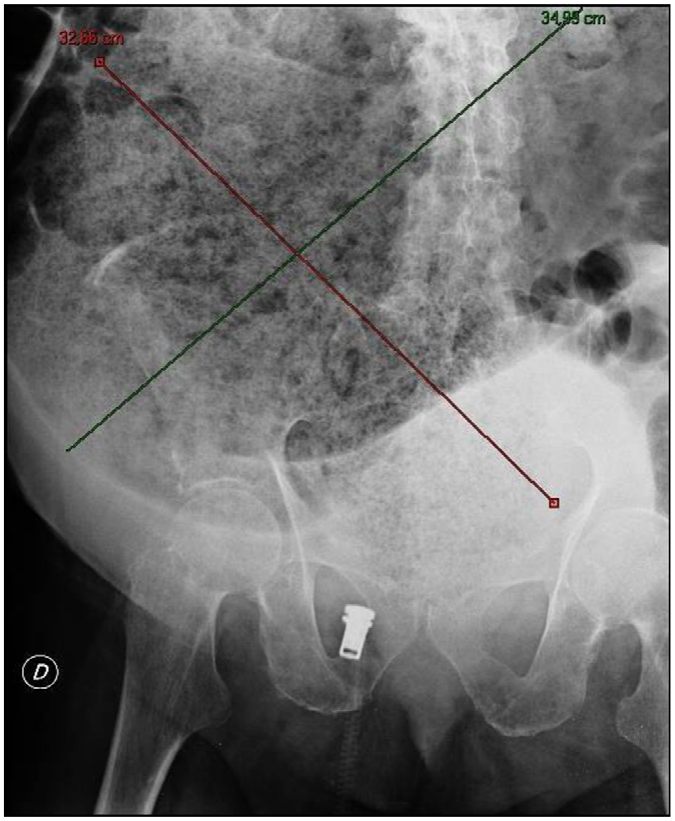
Initial plain abdominal X-ray done in emergency room showing a huge faecal impaction extending from the pelvis upwards to the left subphrenic space and from the left towards the right flank, measuring over 40 cm in length and 33 cm in width.
